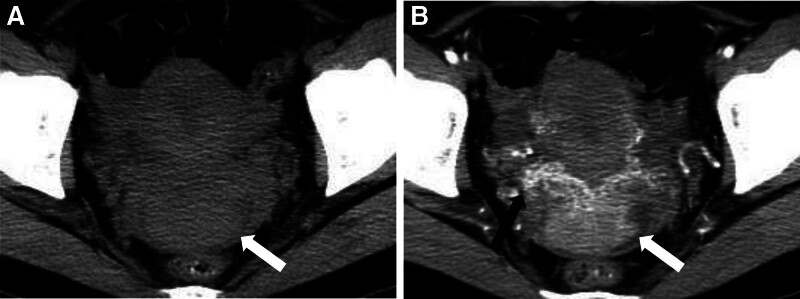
CT image showing clear cell adenocarcinoma of the uterine cervix. (A) Tumor with soft tissue density and a clear boundary (white arrow). The arterial phase image (B) shows obvious enhancement of the tumor (white arrow). Thickened uterine arteries and veins were observed around the bottom of the uterus (black arrow). The venous phase image (C) shows continuous enhancement and (D) enlarged lymph nodes around the bilateral iliac vessels (white arrow).
